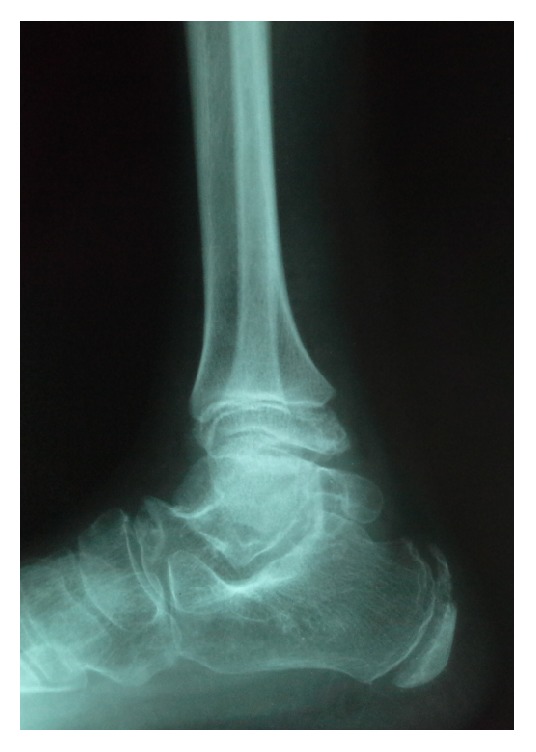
Lateral radiograph of right foot indicating mega os trigonum.
